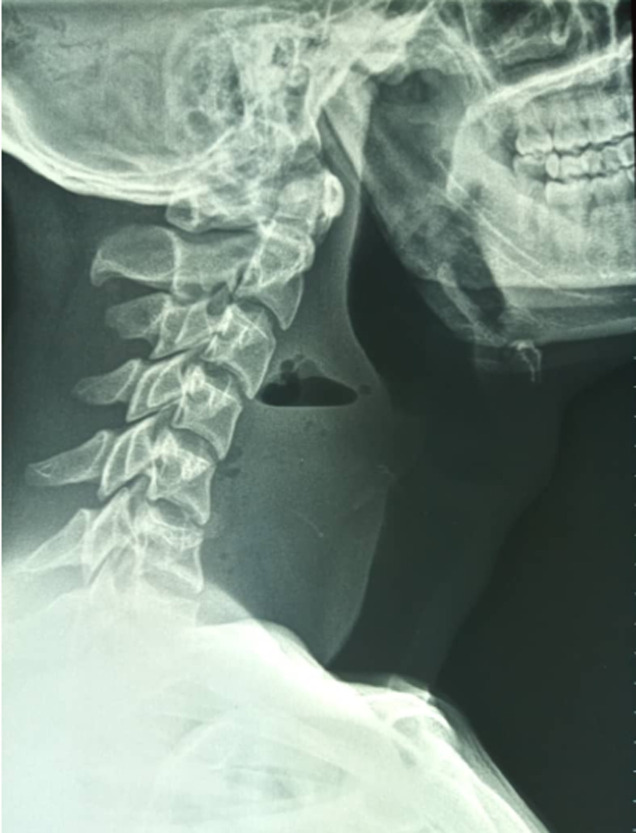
radiographie cervicale profil du 2^e^ patient, montrant une opacité surmontée d´un niveau hydroaérique avec le signe de Miningerode et une opacité linéaire fine, oblique en regard de C4 en faveur du corps étranger
